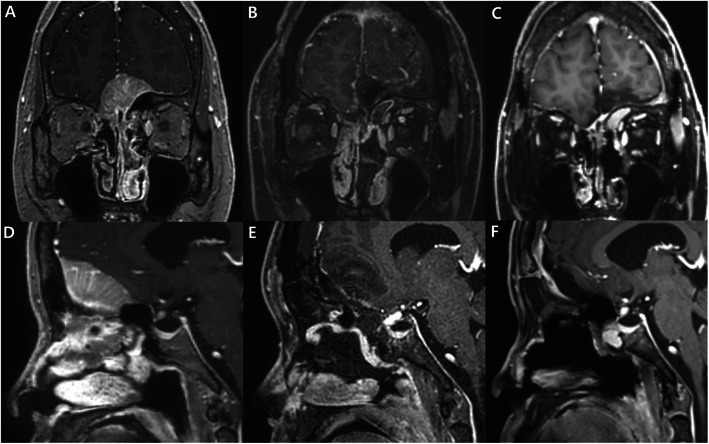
Images from Case 3. A meningothelial meningioma. a Coronal enhanced MRI reveals an extradural mass in the frontal base extending into the upper nasal cavity. d Sagittal MRI shows extensive involvement of the tumor in the upper nasal cavity, but not in the sphenoid sinus. b, e Postoperative contrast-enhanced coronal- and sagittal MRI shows a well-enhanced PNSF that covers the ASB defect. c, f The follow-up coronal and sagittal MRI showed small mucocele in the sphenoid sinus without any symptoms 6 months after the operation. ASB: anterior skull base, PNSF: pedicled nasoseptal flap
